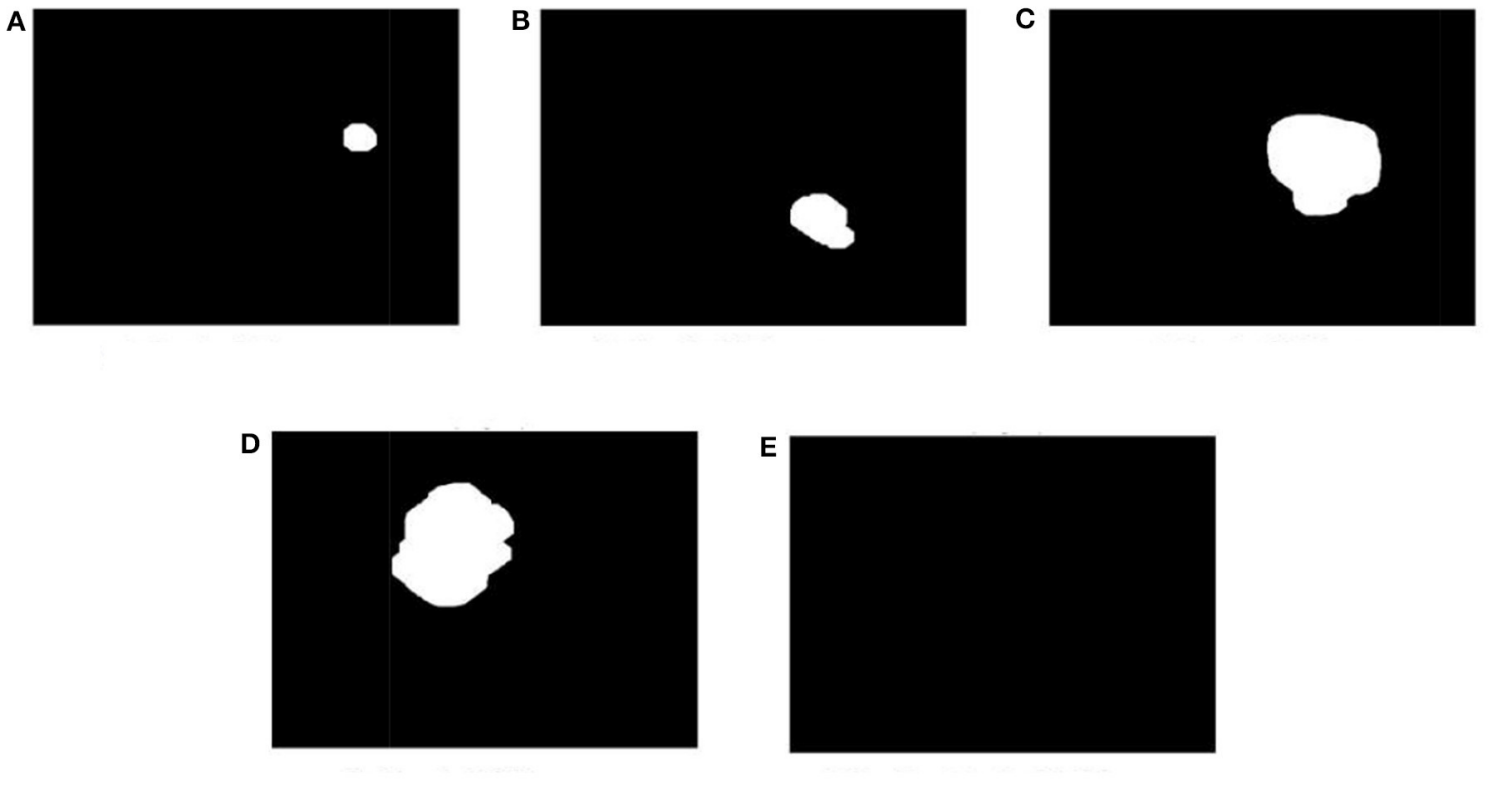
Display a few images from all the labeled folders. (A) Grade I tumor. (B) Grade II tumor. (C) Grade III tumor. (D) Grade IV tumor. (E) Healthy brain MRI image.
